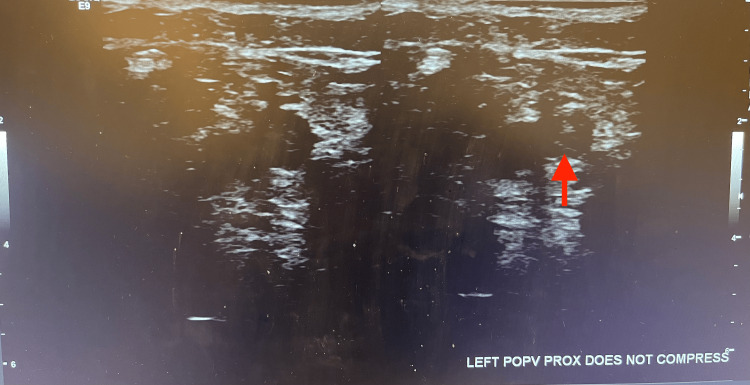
Ultrasound showing noncompressible left proximal popliteal vein DVT DVT, deep vein thrombosis
